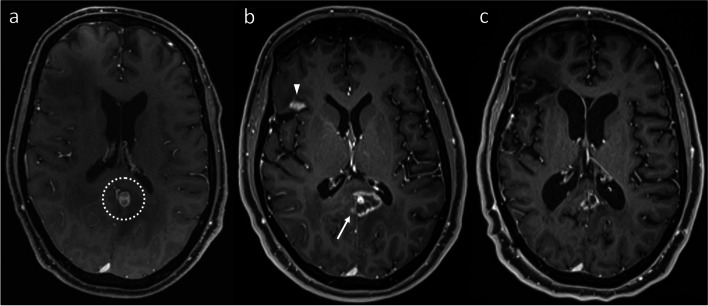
Axial post-contrast T1WI in a patient with an esophageal carcinoma metastasis (a, dotted circle) to the left cingulate gyrus, treated with 20 Gy single-fraction SRS. The lesion enlarged after SRS, with extension to the adjacent corpus callosum. On the 12-month post-SRS MRI (b), a small focus of enhancement developed in the right cingulate gyrus (arrow), discontinuous with the dominant lesion, which allowed a more confident diagnosis of RN. Note the “open ring” enhancement to the left of the falx cerebri; in contrast, if this was contiguous metastatic disease, abnormal enhancement of the falx cerebri would be expected. RN was supported by subsequent follow-up imaging, which demonstrated a change in the shape of the overall abnormality, with some regression of the dominant left-sided component, but further mild enlargement on the right. The appearances stabilized thereafter, and remain stable at most recent follow-up more than 12 months later. The patient also had a right frontal metastasis (b, arrowhead) treated with SRS, but this developed appearances suggestive of PD, prompting resection (which confirmed PD)
